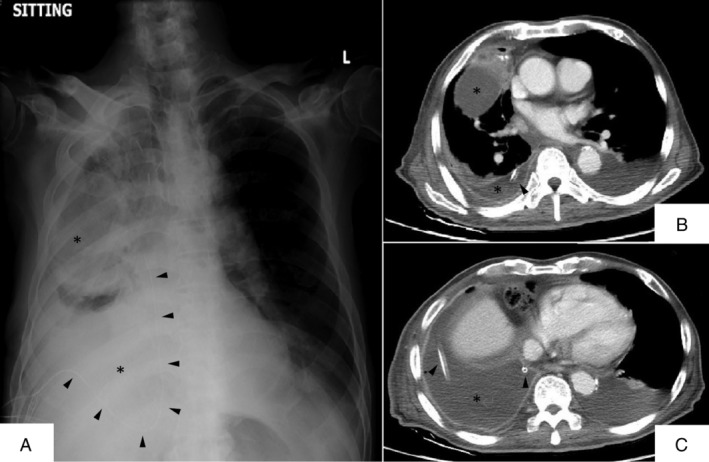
Chest X‐ray (A) and computed tomography images (B and C) showing the indwelling pleural catheter (arrowheads) and loculated right pleural effusion (*).
